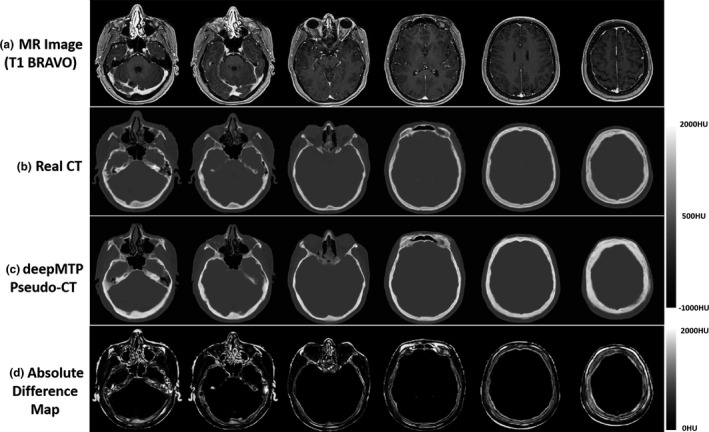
Example pseudo CT images from deepMTP from a 47‐year‐old female patient with right cerebellar tumor. Multiple slices from (a) the input 1.5 T T1 BRAVO MR image, (b) the acquired CT, (c) the pseudo CT generated using deepMTP, and (d) the absolute difference map.
